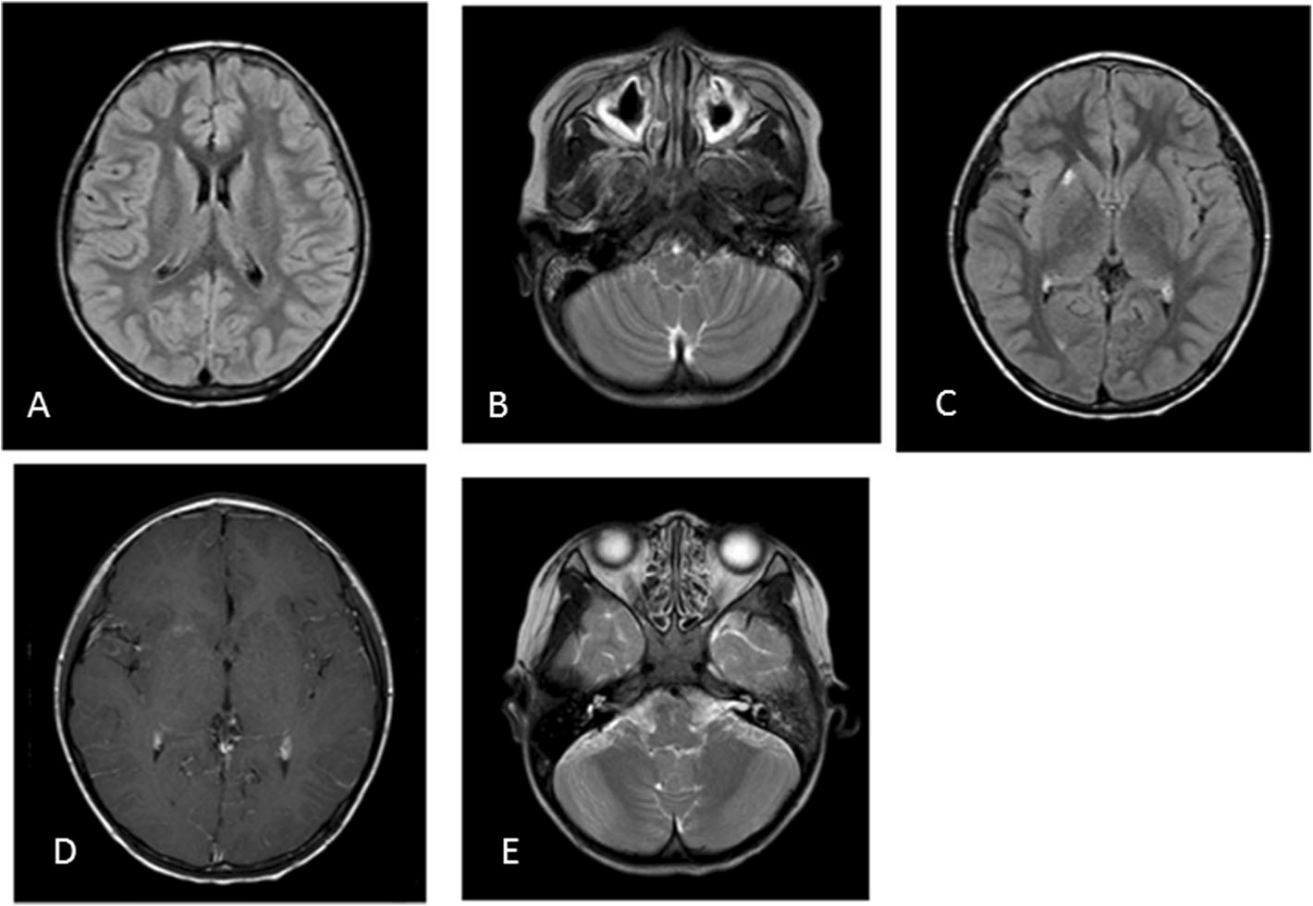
Brain MRI during the two episodes of intracranial infection. A: FLAIR image revealed a little subdural effusion of left frontal, parietal-temporal areas and right frontal area. (1^st^ episode). B: T2-weighted MRI image showedhigh-signal intensity in thickened mucosa of bilateralmaxillary sinus and mastoid process. (1^st^ episode). C: FLAIR image showed high signal on right putamen. (2^nd^ episode). D: Contrast enhanced T1-weighted imagesshowedenhancement of right putamen lesion and bilateral frontal and left temporal lobar meninge. (2^nd^ episode). E: T2-weighted MRI image showedhigh-signal intensity in thickened mucosa of bilateral lethmoidal sinus. (2^nd^ episode)
